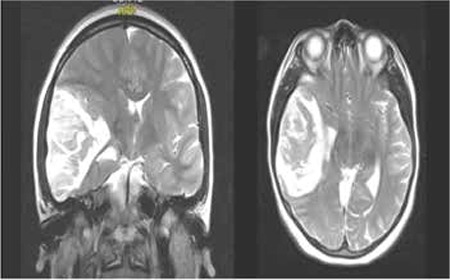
Cranial magnetic resonance images of the patient demonstrate a heterogeneous parenchymal lesion with increased T2 signal intensity in the right temporal lobe causing compression of the third lateral ventricle, basal ganglion, and thalamus with sylvian fissure, sulcal effacement, shifting midline structure, and significant edema.
